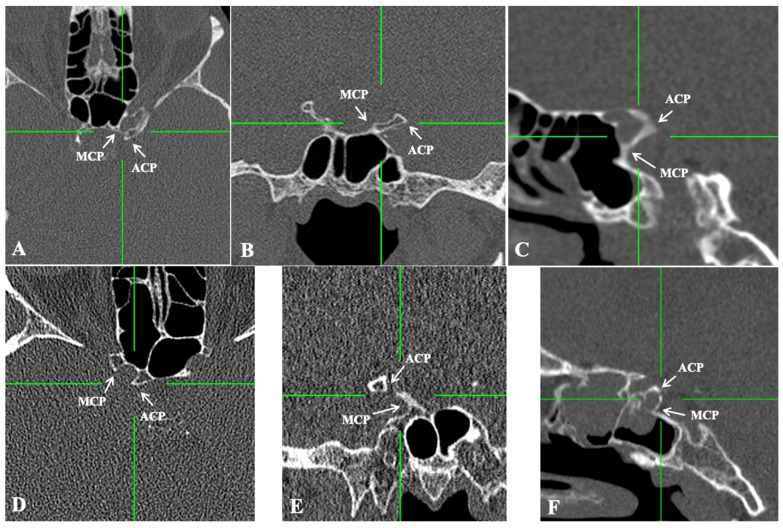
The presence of the caroticoclinoid bar (CCB) in computed tomography scans. (A–C) Axial, coronal, and sagittal reconstructions of a complete CCB. (D–F) Axial, coronal, and sagittal reconstructions of an incomplete CCB. ACP—anterior clinoid process; MCP—middle clinoid process.
